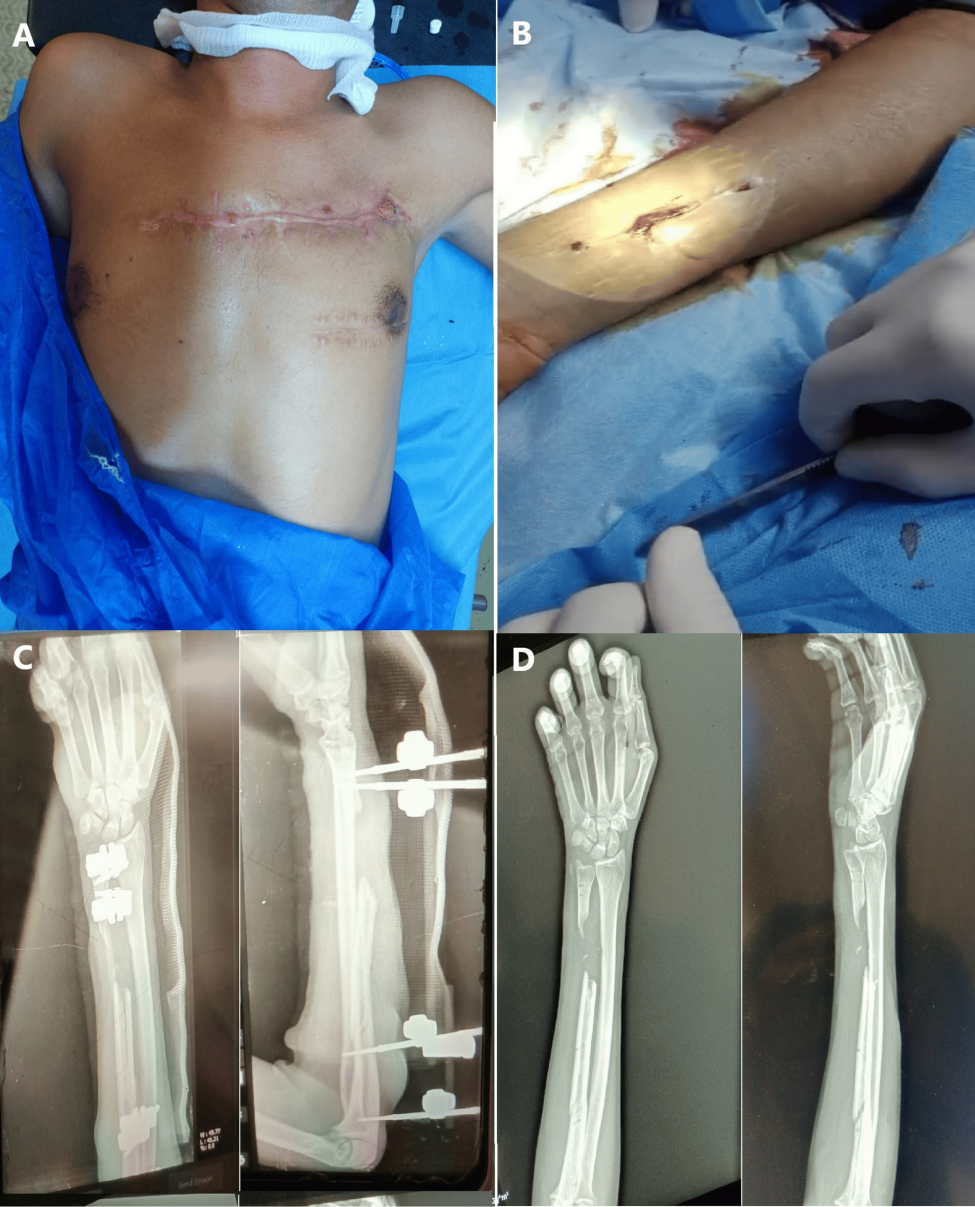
(A) Clinical image of the chest showing two transverse wounds, one at the level of the heart apex, with visible pulsations at the wound site. There is no documentation regarding the extent of the injury, and echocardiogram or chest CT scan was unavailable. A chest X-ray was unremarkable, adding to the complexity of management. As a result, the patient underwent surgery under regional anesthesia. (B) Clinical image of the forearm showing longitudinal wound on the volar aspect. Finger movement caused visible movement of the wound due to tethering of the flexor tendons. (C) Anteroposterior and lateral forearm X-rays showing a segmental ulnar bone fracture with bone loss, stabilized by an external fixator and a splint. (D) X-rays taken after the removal of the external fixator reveal the segmental ulnar bone fracture and associated bone loss.
